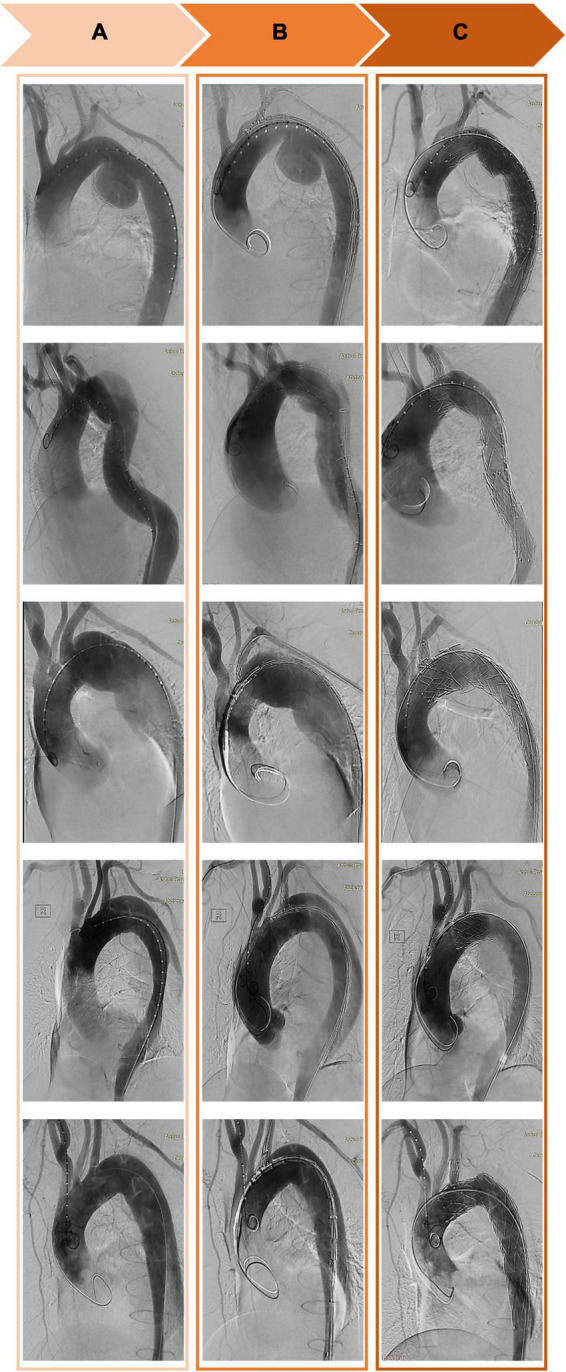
Computed tomography angiography of the thoracic aorta before (A), during (B), and after (C) implantation of a Castor single-branch stent graft.
